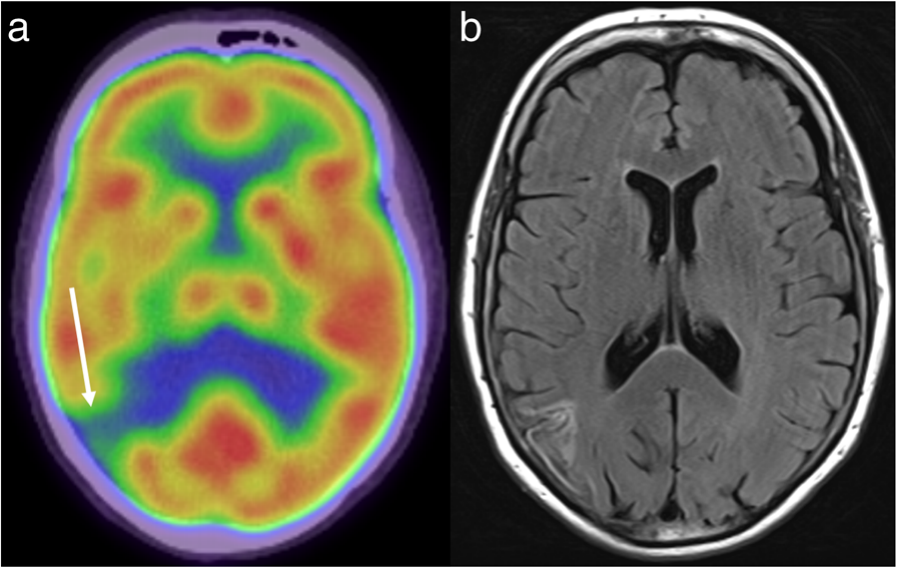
FDG-PET (a) in a patient with metastatic melanoma demonstrates low uptake in the right parietal lobe (arrow). The FLAIR sequence of the corresponding MRI (b) is consistent with a previous infarct rather than a metastasis
